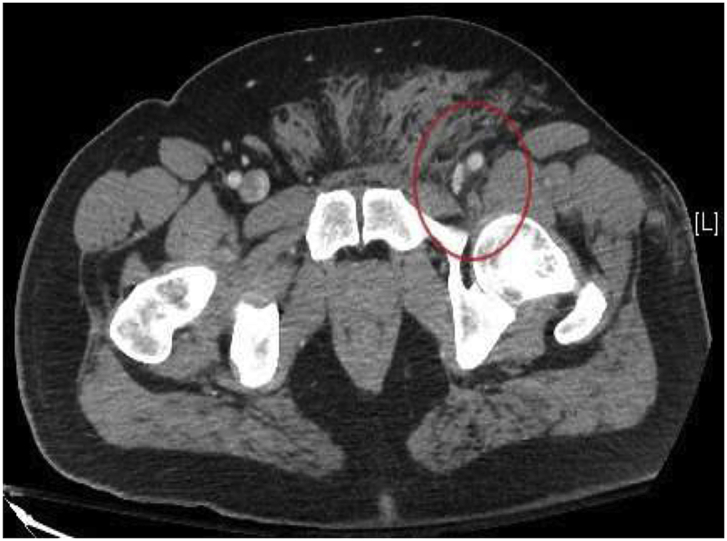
CT axial image of the left common femoral artery and vein just proximal to the traumatic arteriovenous fistula.
